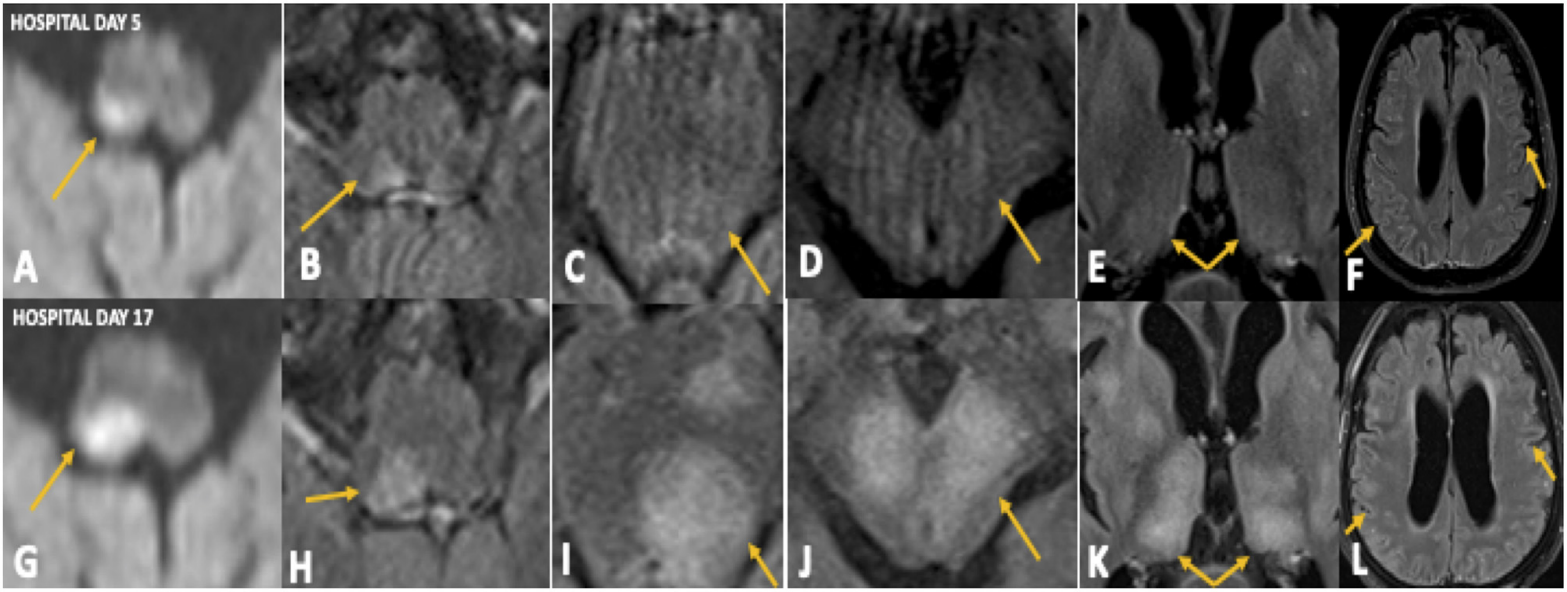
Case 1: Initial MRI brain on hospital day 5 demonstrated diffusion restriction in the right dorsal medulla (A) with corresponding T2 FlAIR hyperintensity (B), faint T2 hyperintensities in the left pons (C), midbrain (D), and thalamus (E), and minimal T2 sulcal hyperintensity without contrast enhancement (F). Repeat MRI brain on hospital day 17 showing significant progression of these abnormalities (G–L).
